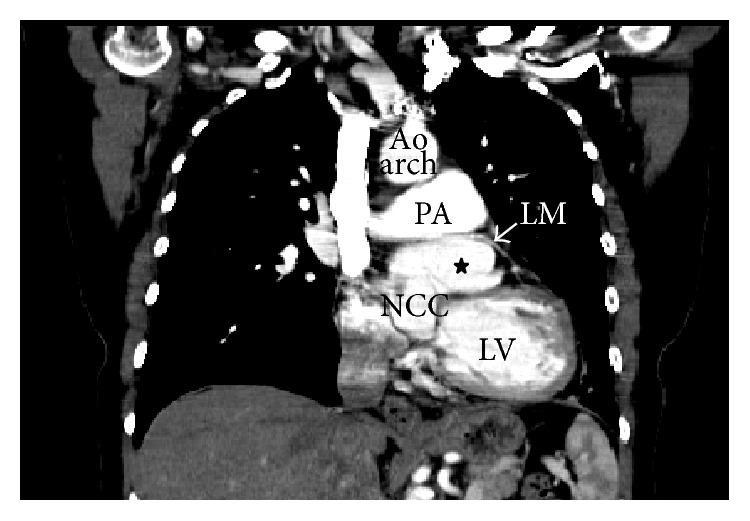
Coronal section of the chest CT shows markedly enlarged left sinus of Valsalva (asterisk). Also note that the course of the left main (LM) coronary artery is displaced superiorly and passing through the dilated sinus of Valsalva and main pulmonary artery (PA). NCC: noncoronary cups, LV: left ventricle, and Ao arch: aortic arch.
